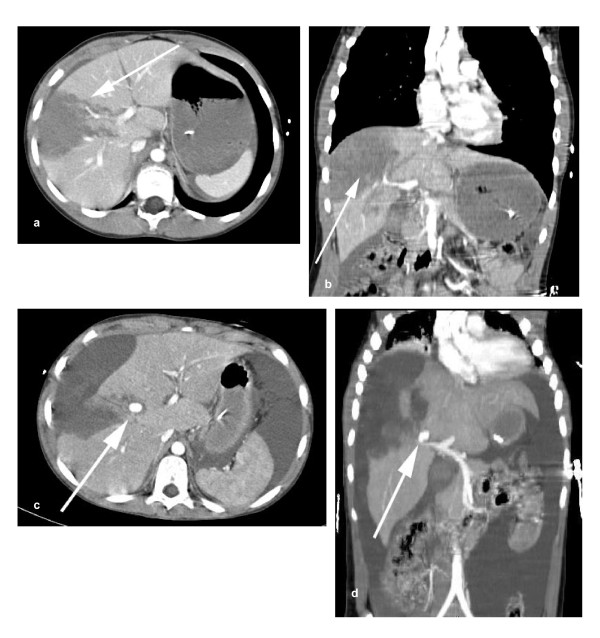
a) Axial contrast enhanced CT of a teenager who sustained a handlebar injury to the abdomen. Large laceration/haematoma (arrow) and no active extravasation. b) Coronal reconstruction demonstrates free fluid around the right lobe of the liver (arrow) and the extent of the laceration. He was managed conservatively initially but deteriorated several days later. c) An emergency CT showed a contrast blush (arrow). d) Maximimum intensity projections demonstrated that the most likely cause was the right anterior portal vein (arrow). At operation (not by our team) biliary peritonitis was found but there was no active bleeding and subsequent hepatic angiography was negative.
